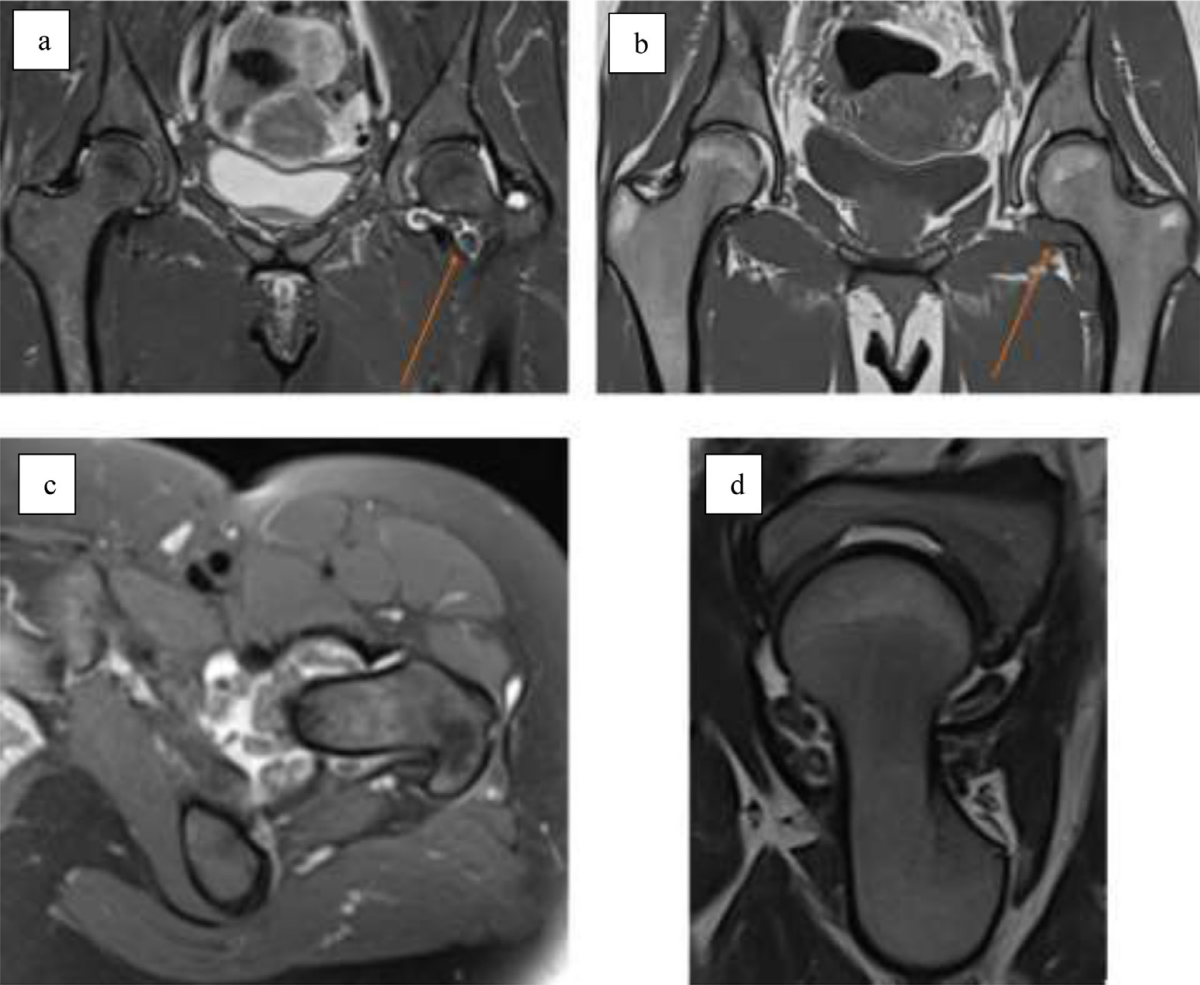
Case 3. 38-year-old female. MRI demonstrates a moderate left hip joint effusion with multiple hypointense intra-articular bodies. (a) Coronal STiR sequence of the pelvis with left hip effusion (arrow), (b) coronal T1 sequence of the pelvis with left hip effusion (arrow), (c) axial PD fat-suppressed sequence of the left hip, (d) sagittal T2 sequence of the left hip.
